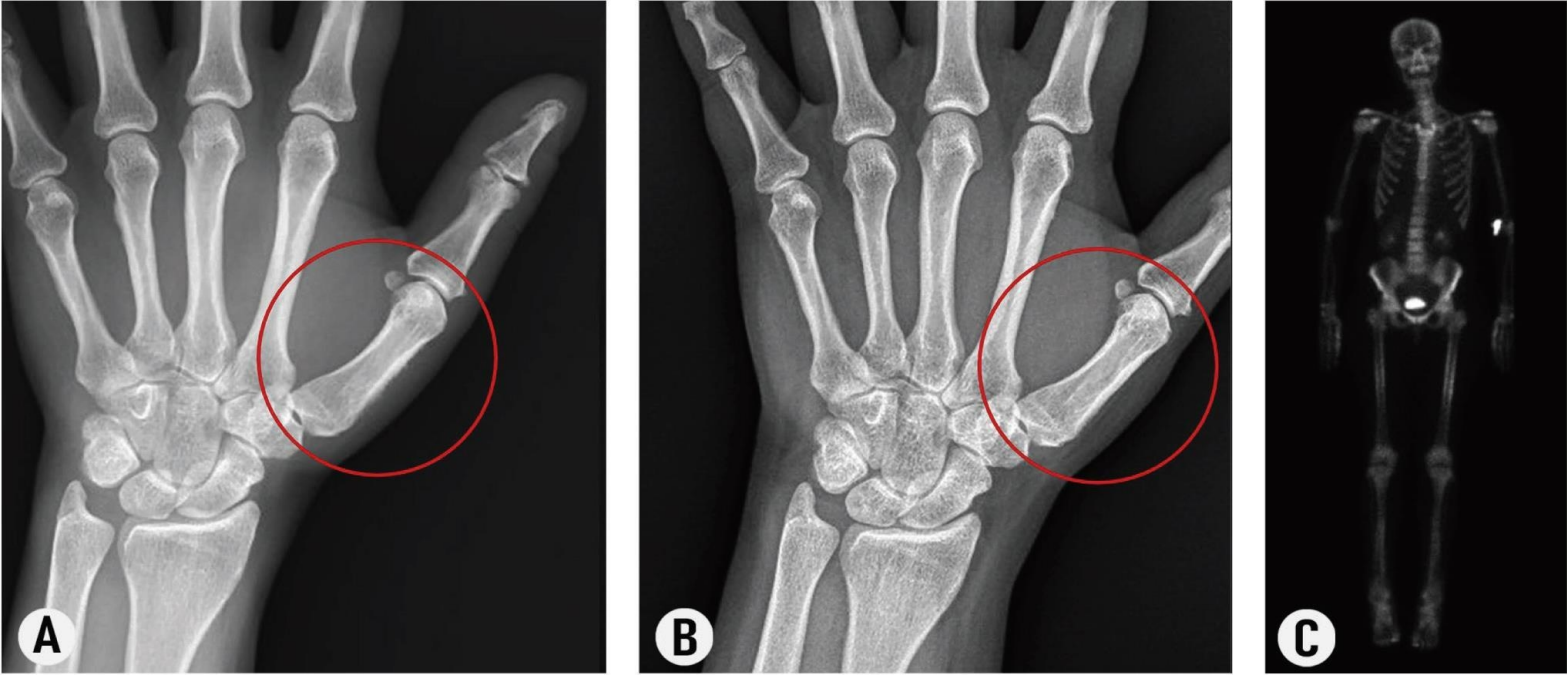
Regression of radiographic and bone scintigraphic findings of thyroid acropachy with teprotumumab: (A) left-hand radiograph showing evidence of periosteal reaction along the radial cortex of the first metacarpal, consistent with thyroid acropachy, (B) left-hand radiograph showing regression of the periosteal reaction following eight 3-weekly infusions of teprotumumab, and (C) 99mTc MDP bone scintigraphy showing bilateral periosteal activities within the midshafts of the femurs, proximal tibiae, and first metacarpals (periostitis).
